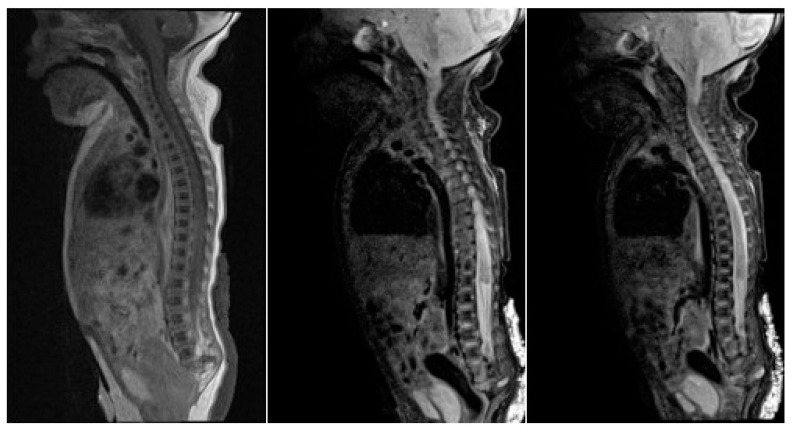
Sagittal MR images show vertebrae down to roughly L5 with no developed sacral elements. The conus medullaris is high at T11, with a truncated, inferior tip.
